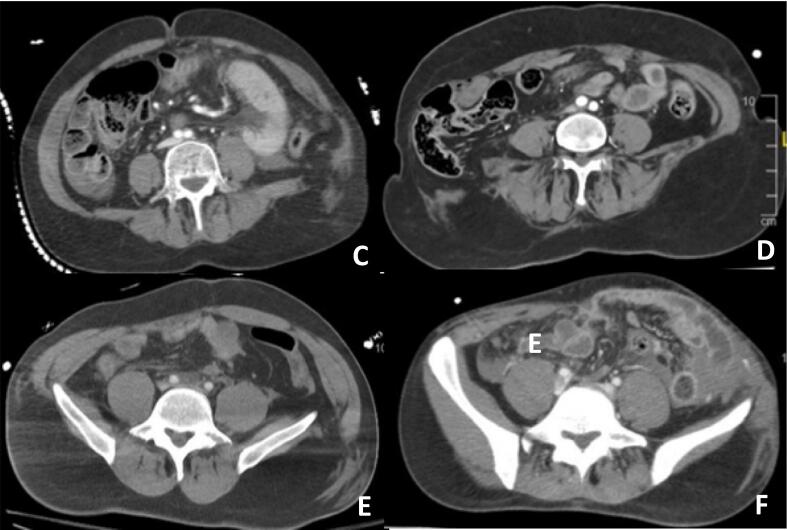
CT scan of patients C, D, E, and F revealing muscular laceration with traumatic hernia.
